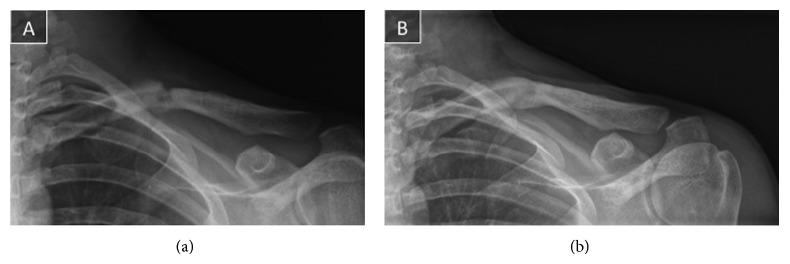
(a) Immediate postoperative radiograph after plate removal 5 months after the first surgery. (b) Six months after plate removal, the patient started contact sports. Complete radiographic consolidation is shown in the front clavicle radiograph.
